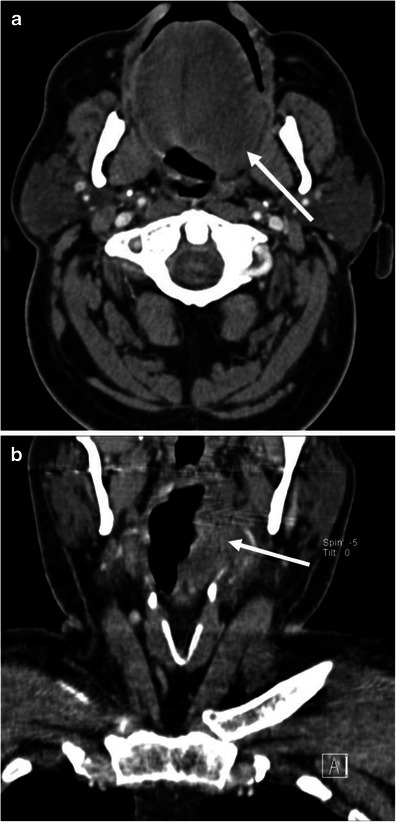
A 62-year-old male with ACE inhibitor-induced angioedema. a The axial image of CE-CT demonstrates asymmetric left-sided tongue oedema (*arrow*). b The coronal reformatted image of CE-CT shows extension of oedema into the left pharyngeal wall (*arrow*)
